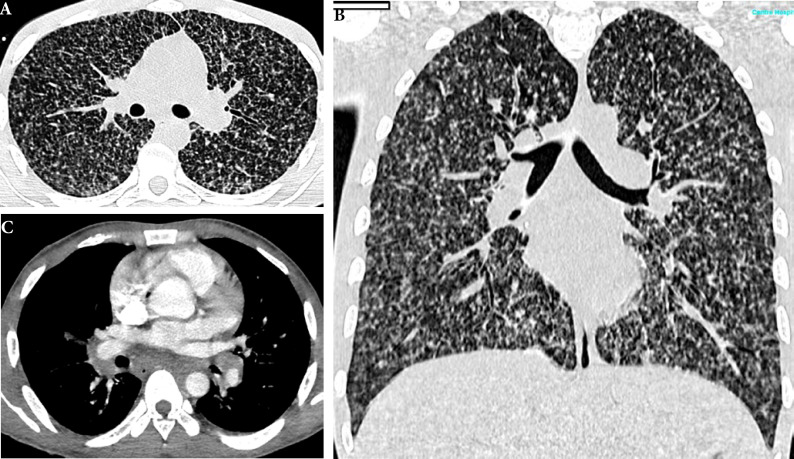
Histoplasmose chez des PVVIH (crédit photo: *M. Zappa)* Légende: A et B. Miliaire hématogène en coupes axiale (A) et coronale (B) sur un scanner thoracique en fenêtre parenchymateuse. C. Adénopathies médiastinales symétriques (hilaires bilatérales et sous carinaire) sur une coupe axiale d'un scanner thoracique en fenêtre médiastinale (crédit photo: M. Zappa) Histoplasmosis in PLWHIV (photo credit: M. Zappa) A and B. Hematogenous miliary in axial (A) and coronal (B) sections of a chest CT scan in parenchymal window. C. Symmetrical mediastinal adenopathies (bilateral hilar and subcarinal) on an axial section of a chest CT scan in mediastinal window
